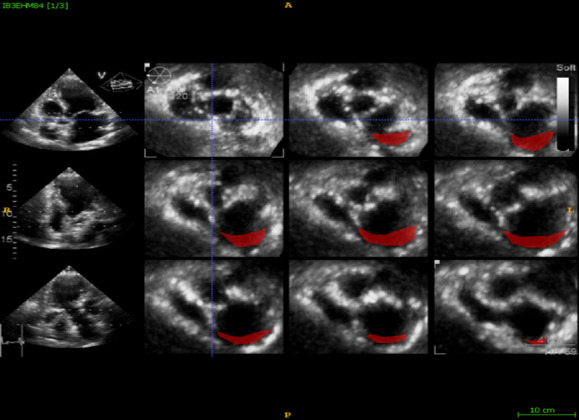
Four-dimensional echocardiography: frame by frame volume measurements of the dilated sinus
